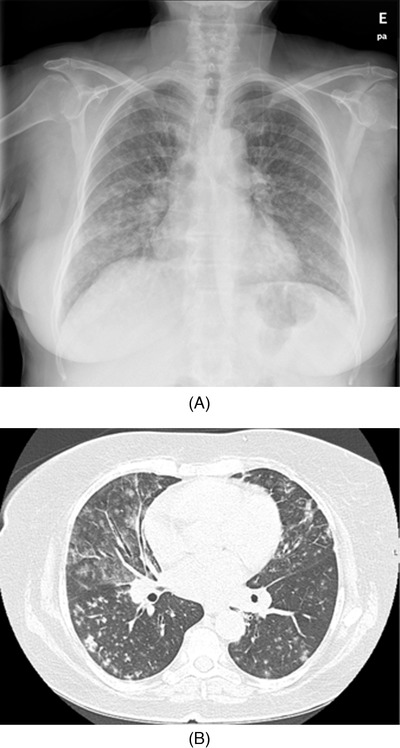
(A) Chest X‐ray shows bilateral interstitial infiltrates. (B) Thorax CT with diffuse bilateral ground glass opacities with micronodules
